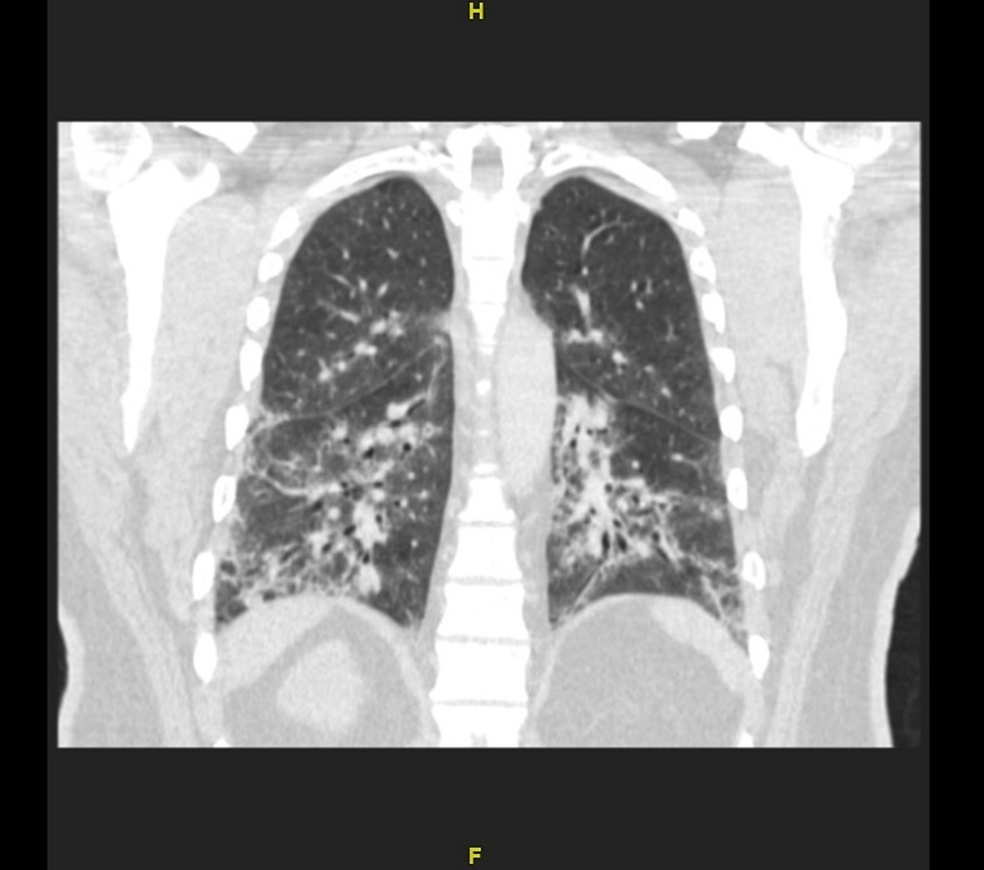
CT of the chest: Interstitial lung disease with bronchiectasis
